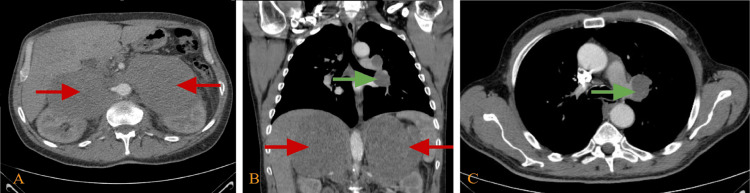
Chest and abdominal CT with intravenous contrast in the venous phase (A) An axial view of an abdominal CT scan showing two large hypodense regular masses in the adrenal glands bilaterally, causing an anterior displacement of abdominal organs with encasing of major vessels; these masses measure 14 x 11 x 17.7 cm and 12 x 10 x 15 cm in anterior-posterior, transverse, and craniocaudal dimensions (red arrows). (B) Coronal view of abdominal and thoracic CT scan showing two large hypodense regular masses in the adrenal glands bilaterally, causing a similar finding (red arrows), and a left hilar lymph node enlargement measuring 3.4 x 2.5 cm (green arrow). (C) Axial view of a thoracic CT scan showing a left hilar lymph node enlargement measuring 3.4 x 2.5 cm (green arrow).
